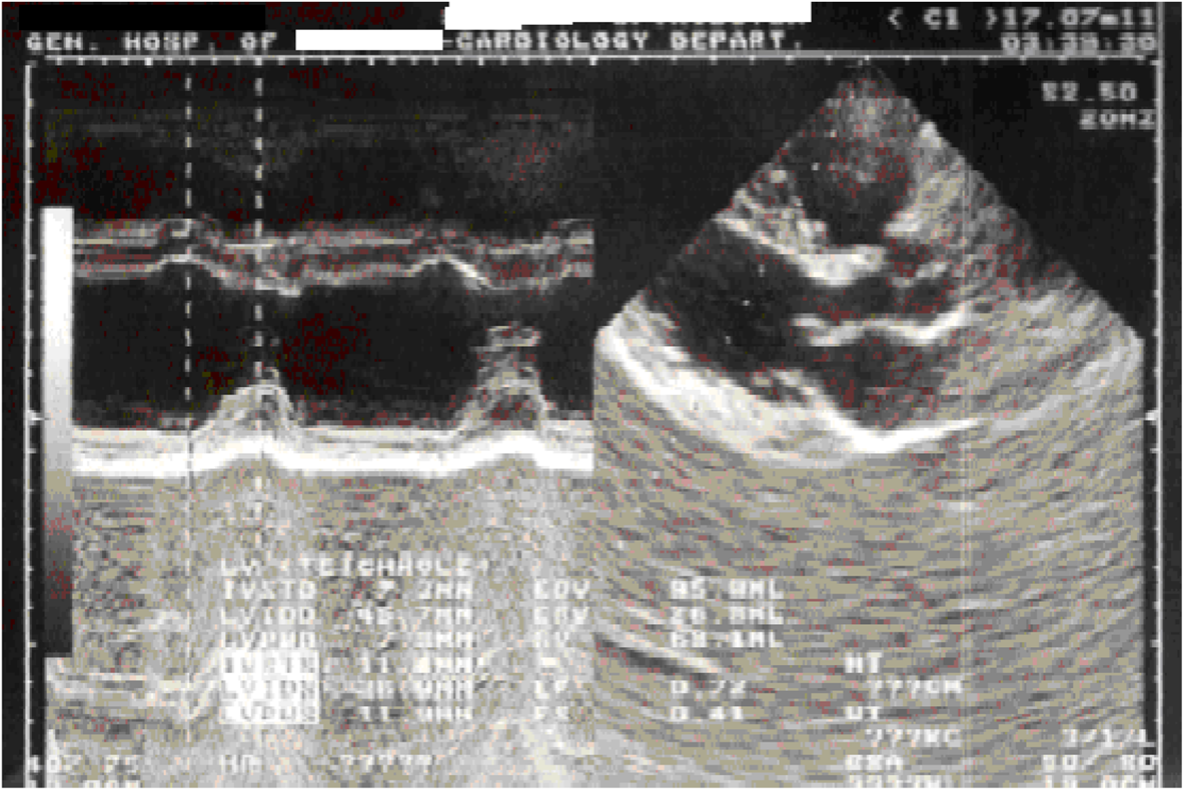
Echocardiogram obtained at the time of admission.
